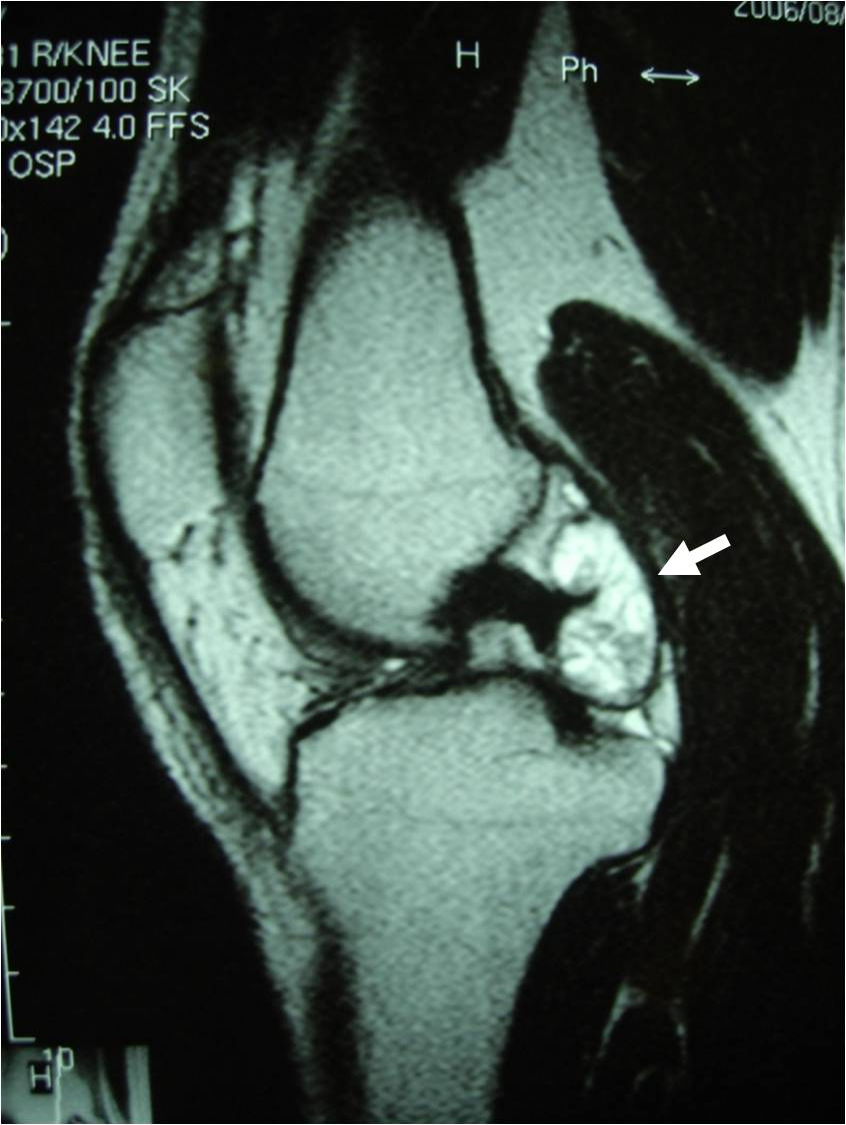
Preoprerative MRI. Magnetic resonance imaging of the right knee showed a 2.5 cm tumor located in the dorsal area of the posterior cruciate ligament (arrow). The tumor exhibited a fluctuating high and low signal intensity with low signal septa around the tumor on T2-weighted images.
